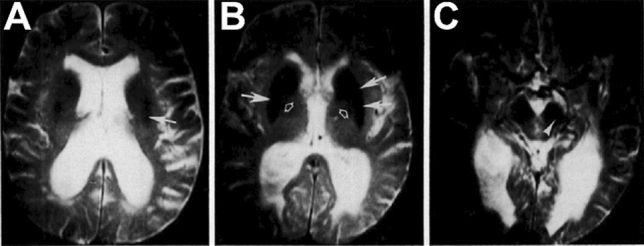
Early T2-weighted magnetic resonance imaging using a 1.5 T field strength of iron in a multiple system atrophy. White arrows in a, b denote decreased signal intensity, indicating iron deposits, in the putamen and caudate nucleus compared to the globus pallidus (open arrows, (b)). Hypointensity marked with the white arrowhead (c) was interpreted as indicative of specific iron accumulation in the substantia nigra pars compacta. Reproduced from Drayer et al. 1986a with permission from the Radiological Society of North America (copyright 1986)
